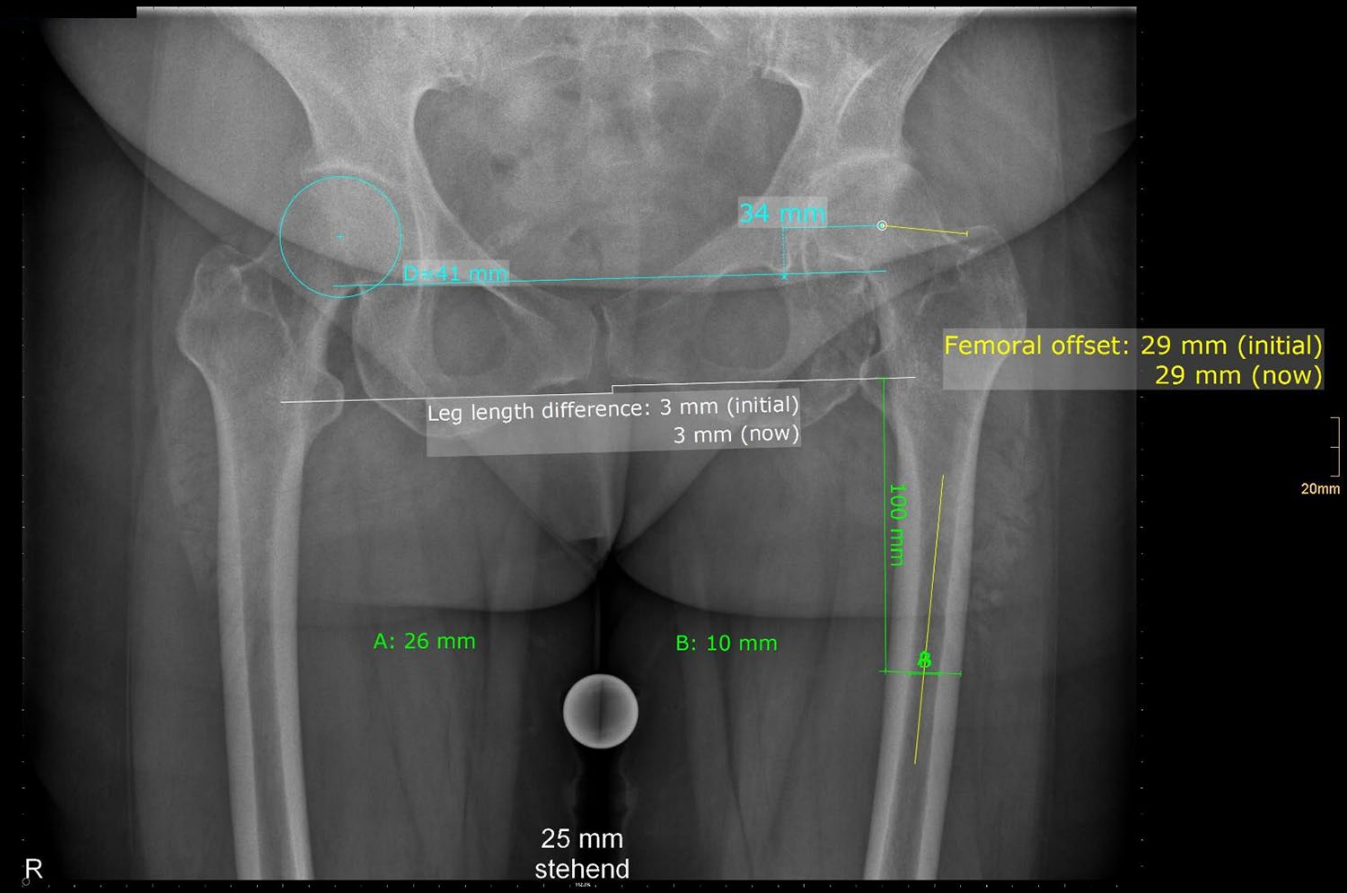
Preoperative X-ray with measurement of femoral offset (FO), acetabular offset (AO), leg length difference (LL) and Cortical Index (CI)
